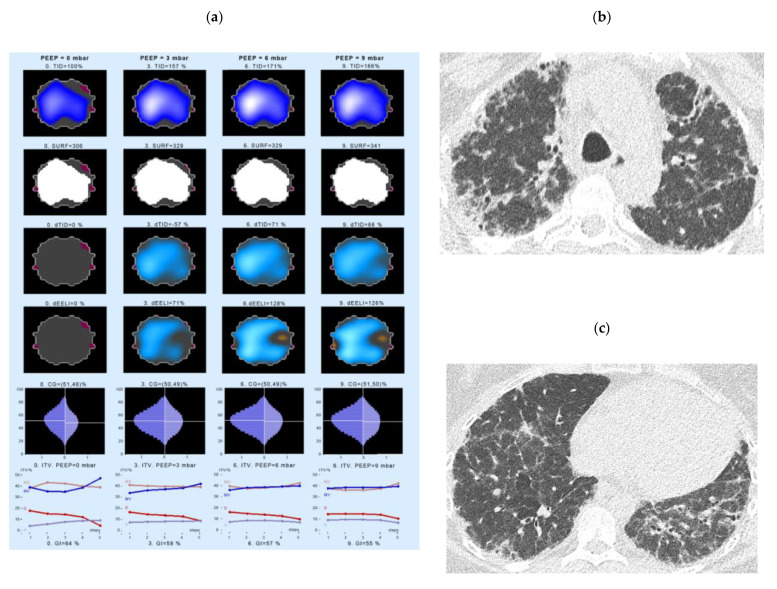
Changes in regional lung ventilation under increasing PEEP in IPF patient #8. Left panel (a): Spatial distribution of TID, SURF, dTID, dEELI in the EI tomogram at a PEEP of 0 (left), 3 (2nd left), 6 (3rd from left) and 9 levels (right column). The lowest panel indicates the intratidal gas distribution (ITV) for four anatomical areas during one respiration. Given are the distribution of ventilation into the ventral (V), dorsal (D), medio-ventral (MV) and medio-dorsal (MD) regions of the lung. The X scale (1–5) shows the entire inspiration, divided into 5 steps. Right panel (b,c): The respective high resolution CT picture corresponding to the ROI of EIT measurement is shown. Abbreviations: CG—center of gravity of ventilation distribution; dEELI—difference of end-expiratory lung impedance over time; ITV—intratidal gas distribution; PEEP—positive end-expiratory pressure; SURF—surface of ventilated areas; TID—tidal impedance distribution; dTID—difference of TID over time.
